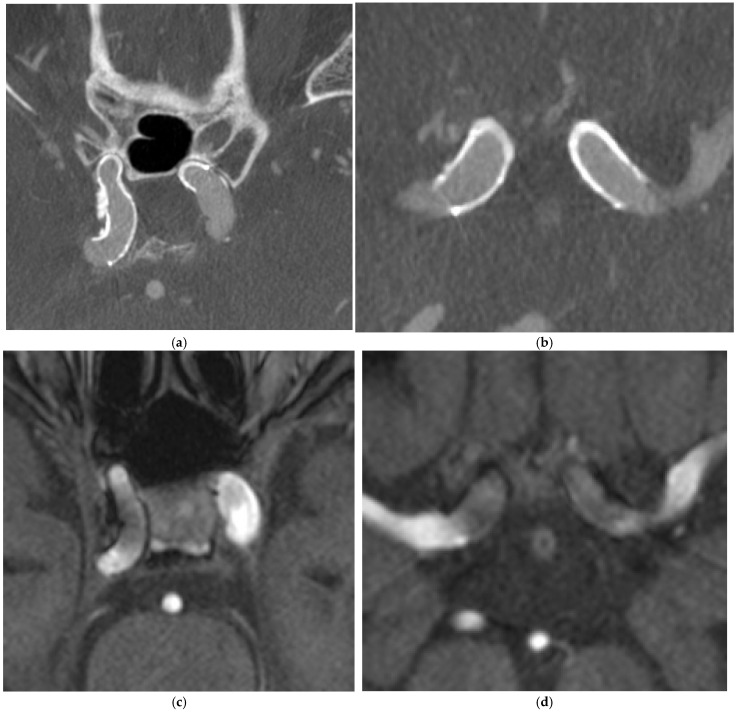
Flow diverter deployment for bilateral ophthalmic internal carotid artery (ICA) aneurysms. (a,b) IV CBCT with MAR showed stents well apposed to vessel wall, well-expanded stent ends and patent bilateral ICAs. Wall calcifications along stented segment of right ICA are also evident. (c,d) MRA showed bilateral stents with susceptibility artifacts. Stent apposition, stent struts, patency of parent vessel and vessel wall status of stented segment are compromised.
